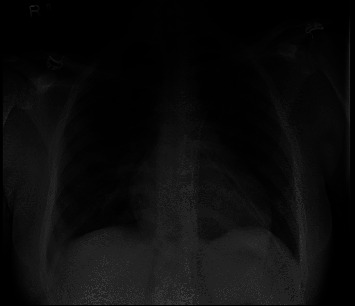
Antero-posterior (AP) view chest X-ray showing bilateral infiltrates.
